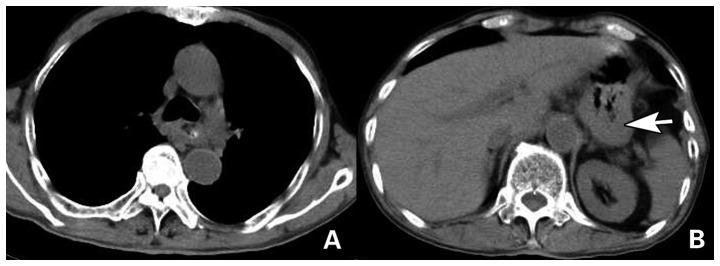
Computed tomography (CT). (A) Pectoral CT reveals a large, heterogeneous tumorous formation obturating the esophageal lumen, with no enlarged lymph node observed, located in the same plane as the lower tracheal bifurcation. The value of CT is ∼78 Hu. (B) The abdominal CT reveals the nodular increased thickness of the gastric antral wall and the narrowing gastric cavity.
